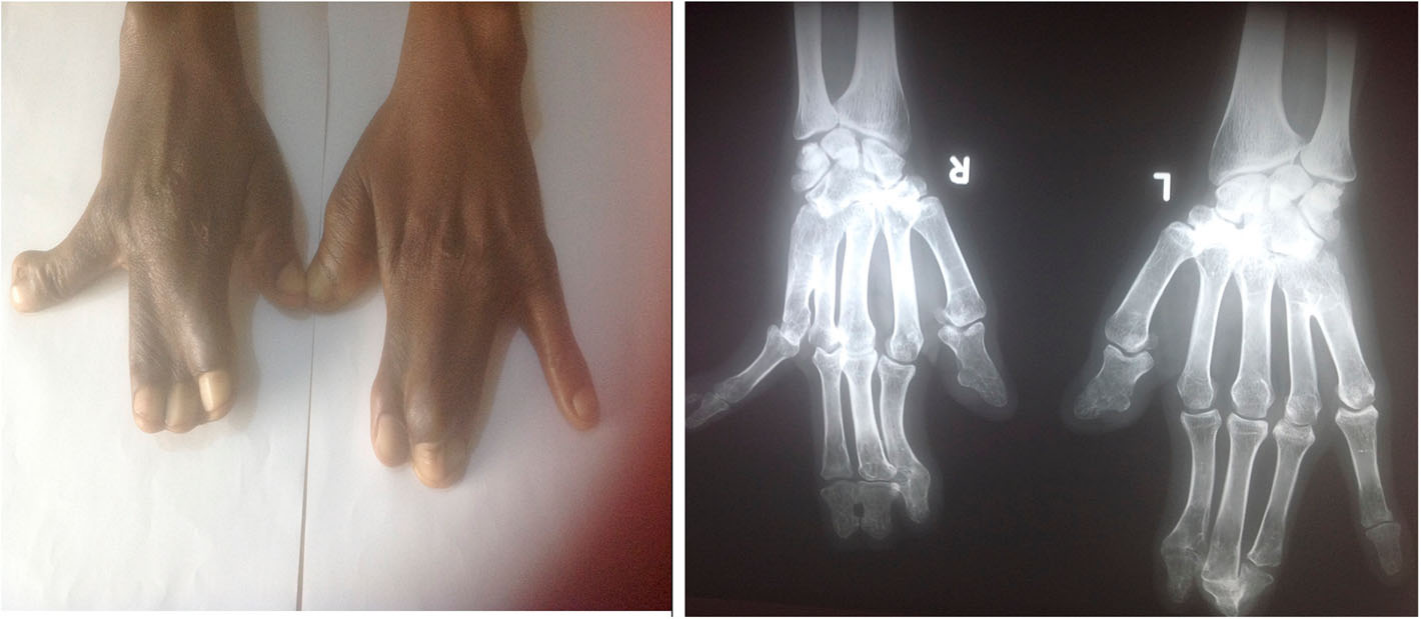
Picture and X-ray of the hands
